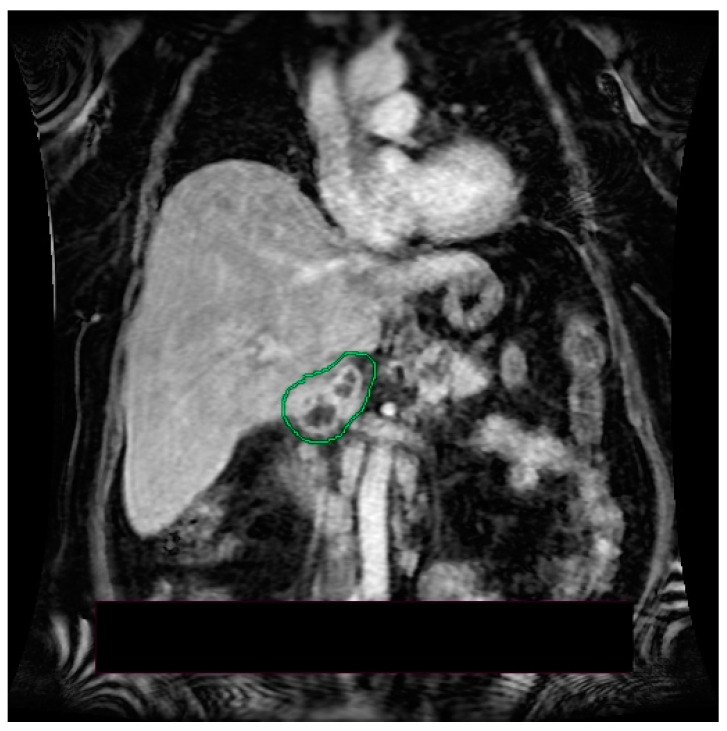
MRI—T1 post contrast sequence, coronal imaging plane: inhomogeneous cephalopancreatic lesion.
